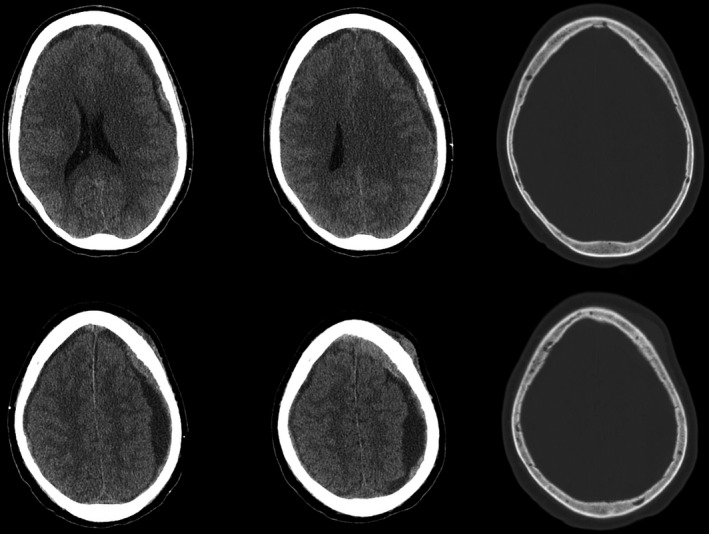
CT axial images of the head demonstrating left greater than right acute on chronic subdural hematomas with midline shift and mass effect without evidence of fracture or bony abnormalities.
